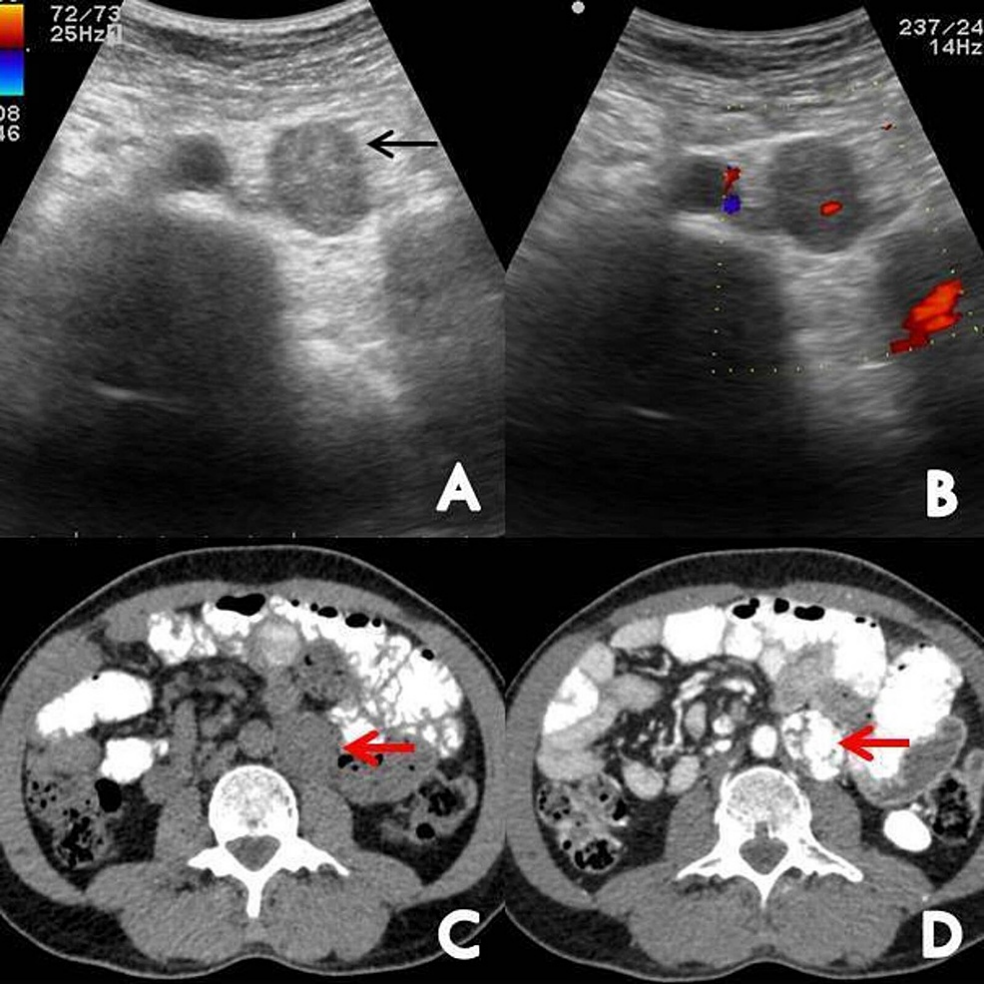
Ultrasound showed an oval heterogenous mass lesion seen in the left para-aortic region (black arrow) (A) with prominent internal vascularity on color Doppler (B). Plain (C) and contrast-enhanced (D) axial images of the abdomen showed a well-defined hypodense solid lesion in the left para-aortic region (red arrows) with avid post-contrast enhancement.
